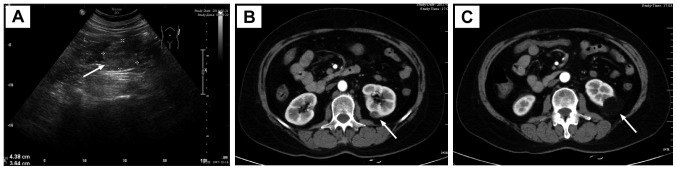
Preoperative imaging showing two renal masses in the lower pole of the left kidney. (A) Renal masses (arrow) were detected by ultrasound. (B and C) Renal masses (arrow) were detected by CT.
